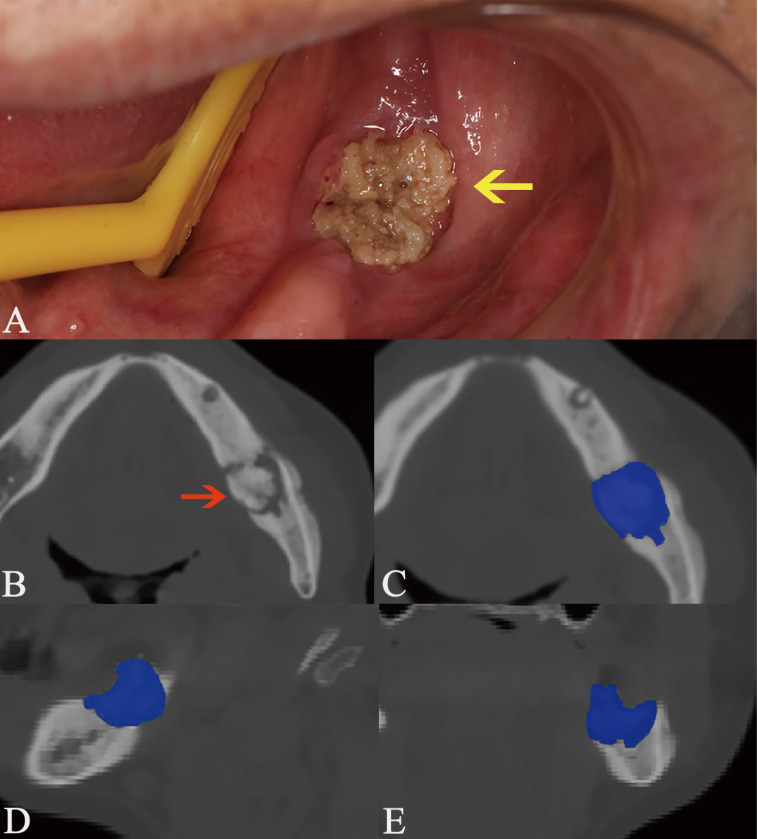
Osteolytic lesion containing sequestrum. The lesion is pointed out by the arrow in intraoral photograph (A) and axial plane (B). The manual segmentation of CBCT images in axial (C), sagittal (D), and coronal plane (E) is highlighted as blue area.
